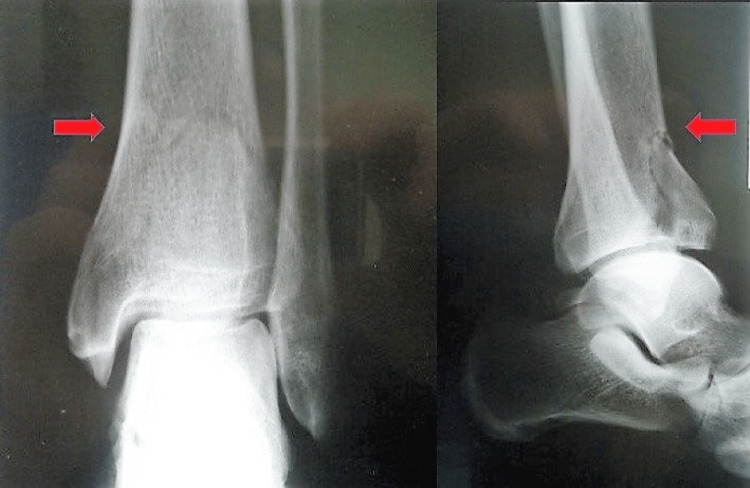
Anteroposterior and lateral X-ray indicating the uncommon type fracture of the anterior margin of the distal tibia (red arrow)
